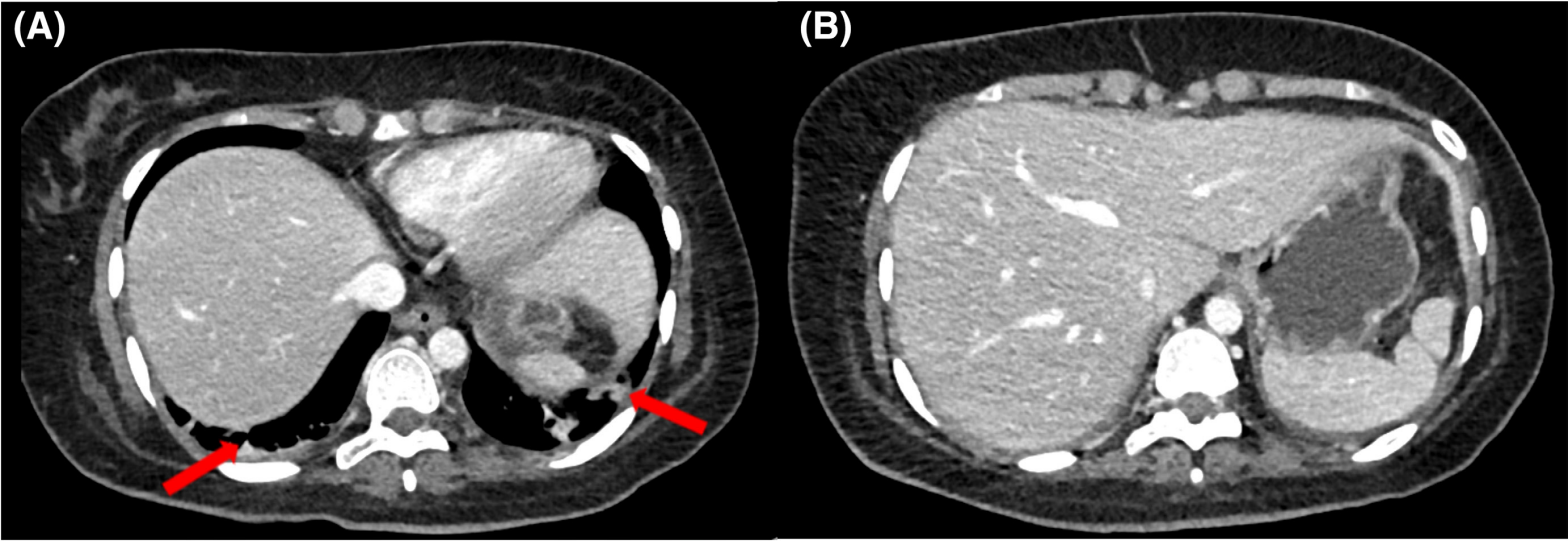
CT Abdomen with contrast, axial cuts (A) lower cut of thorax showing bilateral basal atelectasis with segmental collapse and complete resolution of the bilateral pleural effusion. (B) Total resolution of the previously seen micro abscesses in the left lobe of the liver was also noted.
